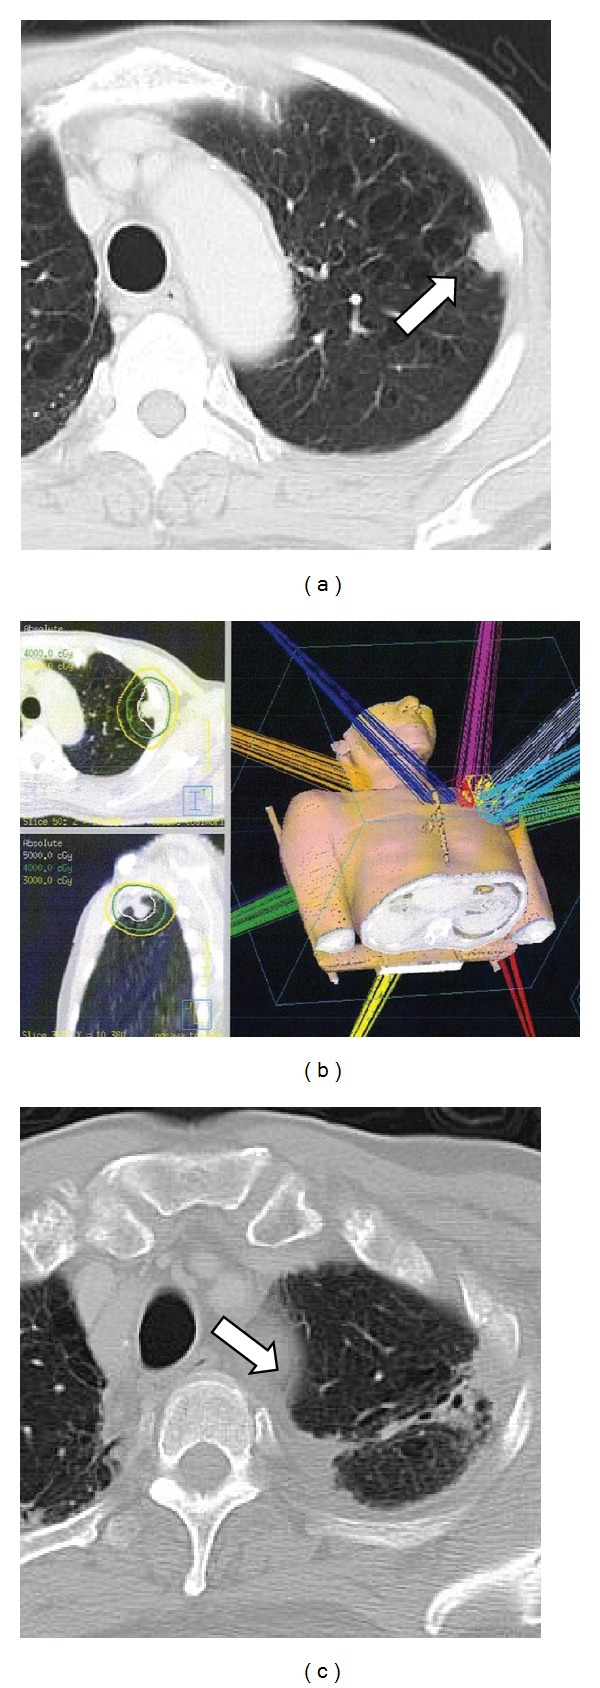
(a) CT of the left lung metastasis (arrow) before SBRT. (b) Dose distribution made with 10 noncoplanar beams for SBRT. The isodose lines shows total doses (in 4 fractions) of 50 Gy, 40 Gy, and 30 Gy, respectively, from innermost area. (c) CT at 2 years after SBRT, showing partial response of the lesion (arrow) with focal radiation fibrosis.
